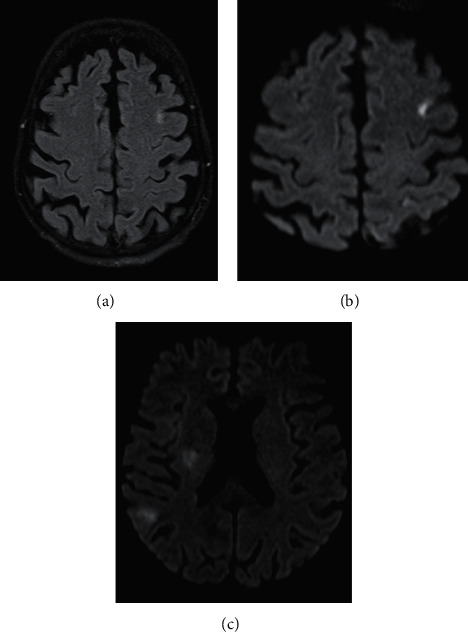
Bilateral ischemic lesions: at the left cortical frontal lobe in FLAIR and diffusion hypersignal (a, b) and in the right parietal and lenticular in diffusion hypersignal (c).
